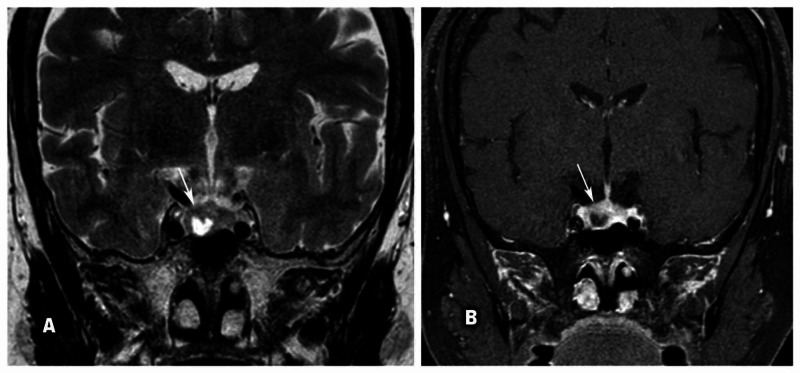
MRI images showing pituitary adenoma Coronal T2-weighted image (A) and early dynamic contrast-enhanced T1-weighted image (B) show heterogenous lesion in the right half of the pituitary gland (~9 mm), showing central non-enhancing T2 hyperintense area and eccentric nodular enhancement (hypoenhancement relative to the rest of the pituitary gland), likely pituitary microadenoma.
